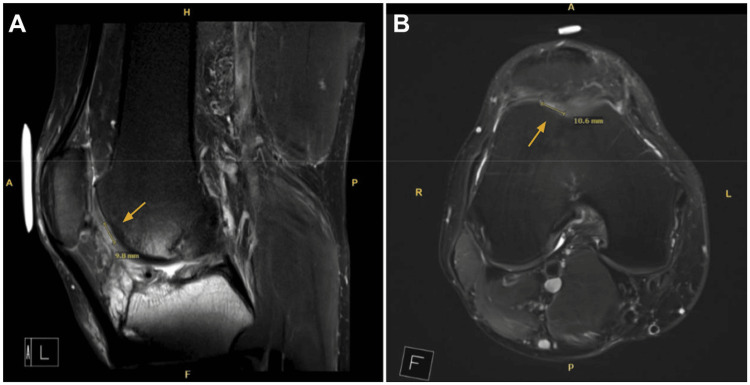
Preoperative magnetic resonance imaging of the right knee in a patient with a focal full-thickness chondral defect of the lateral trochlea. (A) Sagittal image with orange arrow indicating the defect, measuring approximately 1 cm in the anteroposterior dimension. (B) Axial image with orange arrow indicating the defect, measuring approximately 1.1 cm in the transverse dimension. Yellow labels denote anatomic orientation (A, anterior; H, head; F, foot; P, posterior; R, right; L, left).
